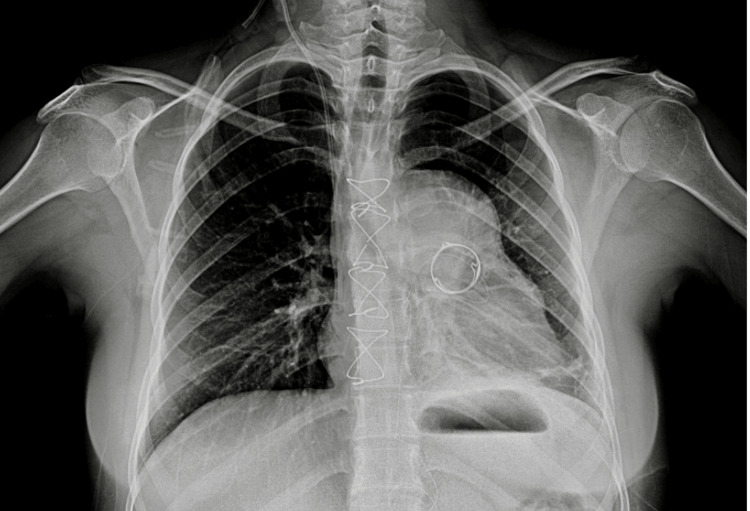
Postoperative chest radiograph demonstrating appropriate positioning of the pulmonary valve prosthesis, with improved pulmonary congestion compared to the preoperative study.
